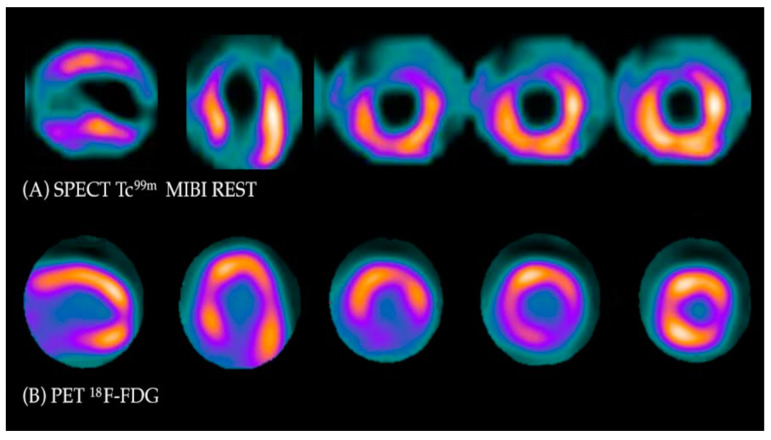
Myocardial viability imaging: Mismatch pattern, viable hibernated myocardium. (A) Myocardial perfusion imaging with MIBI-Tc^99m^ SPECT: heart scans showing lack of tracer uptake in the apical region and apical segments of the anterior wall (left anterior descending coronary artery territory). (B) ^18^FDG viability PET. ^18^FDG uptake is visible in the area of the perfusion deficit, ruling out scarring and confirming viability in this region.
